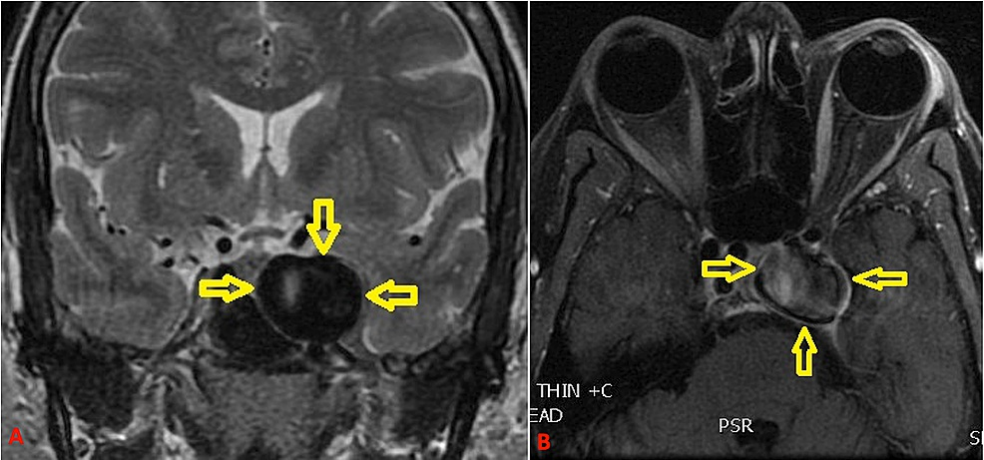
MRI obtained for clinical presentation of left cavernous sinus syndrome. Coronal T2-weighted (A) and axial T1 fat-suppressed (B) images demonstrate the lesion in the left cavernous sinus with flow void. MRI, magnetic resonance imaging
